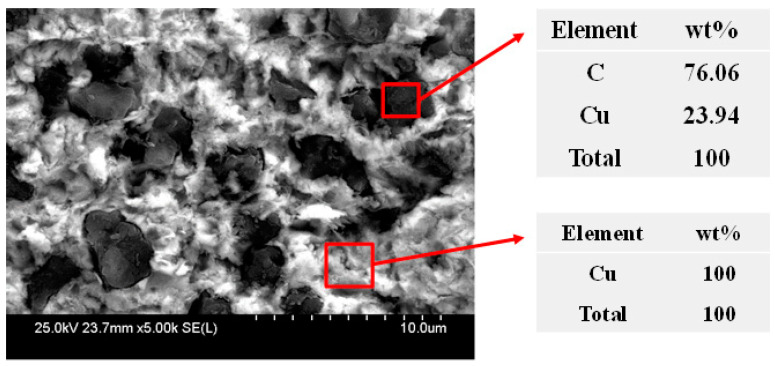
Energy dispersive analysis of X-ray (EDAX) results after lapping.
